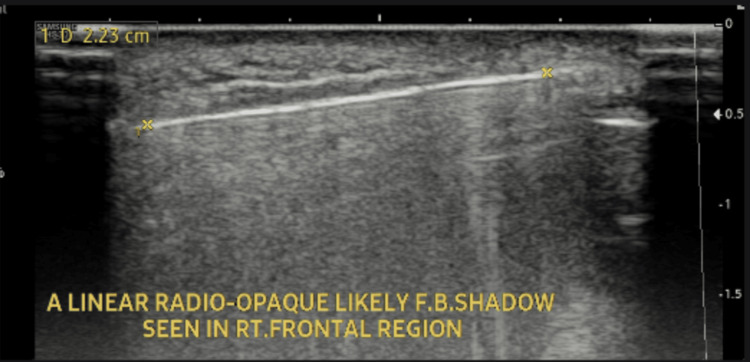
Ultrasound image with the thin linear hyperechoic area F.B.: foreign body; RT.: right
